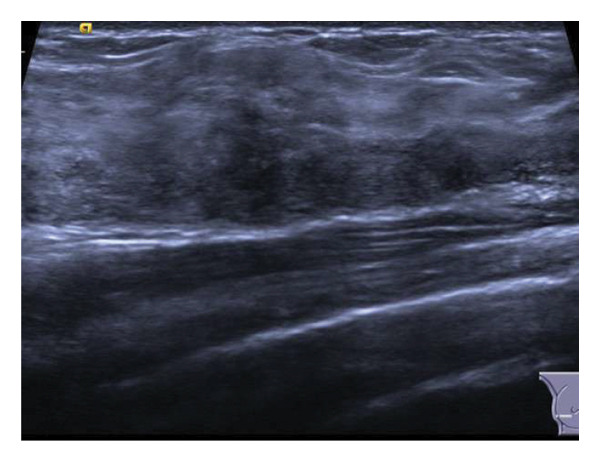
A 35‐year‐old patient with diagnosis of type 1 diabetes mellitus presented for further evaluation of a hypoechoic area with posterior acoustic attenuation at breast ultrasound, corresponding to a clinically evident swelling in the lower outer quadrant of the right breast.
